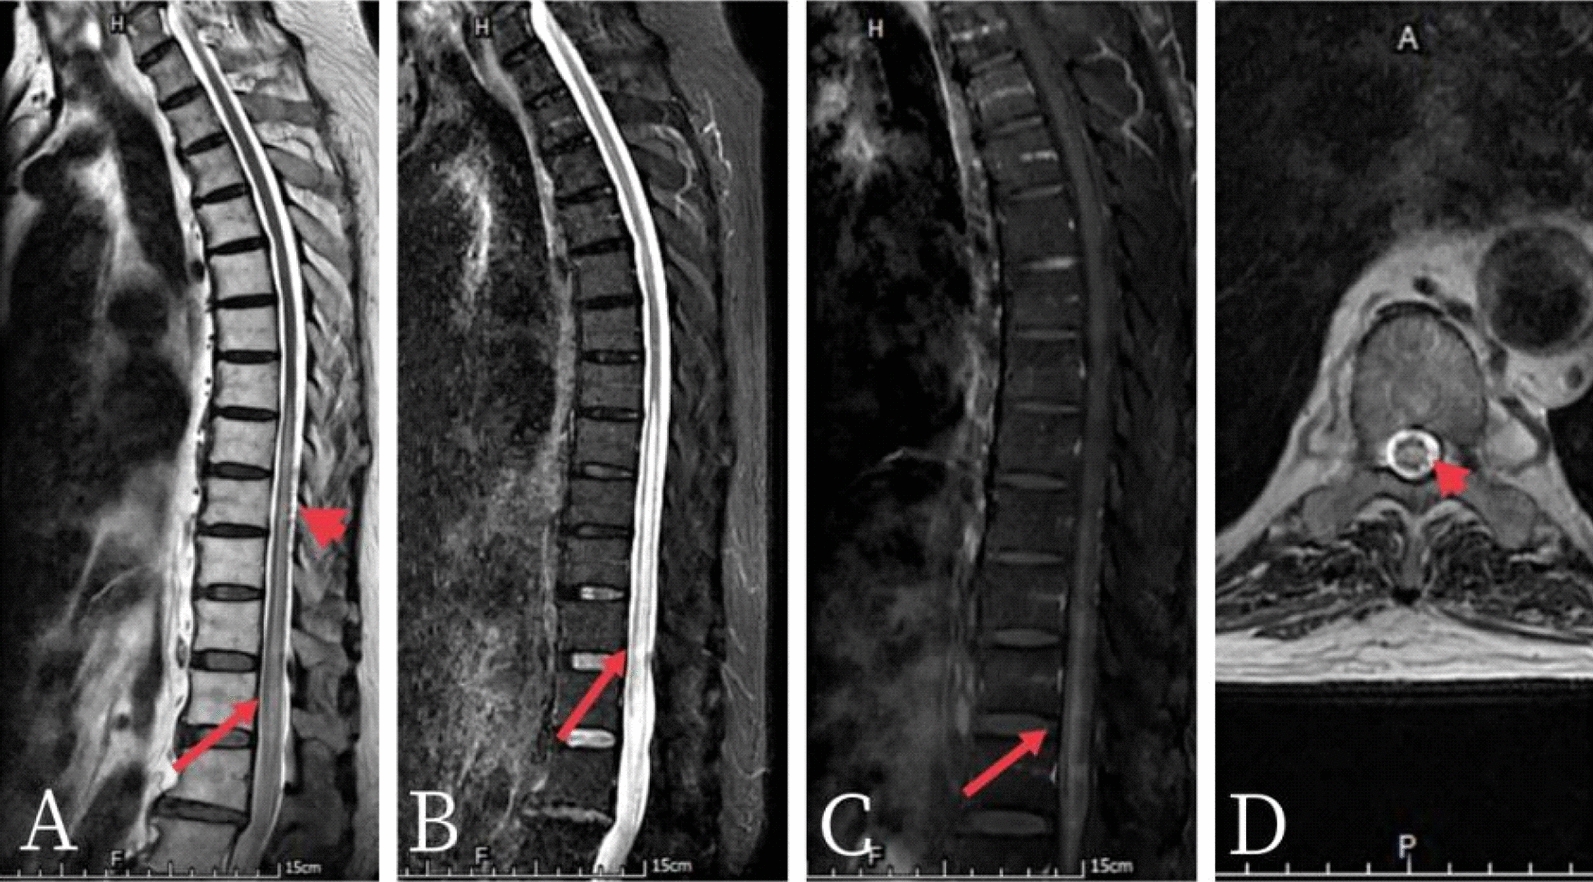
Enhanced MR image of the thoracic vertebra after 4 weeks of rehabilitation training A-D show the T2, STIR, enhanced sequence and axial scans, respectively. The multiple abnormal signals and linear enhancement signals (long red arrow) in the thoracic spinal cord were similar to those in the previous examination. The empty shadows of vascular flow (short red arrows) were slightly decreased compared to before
